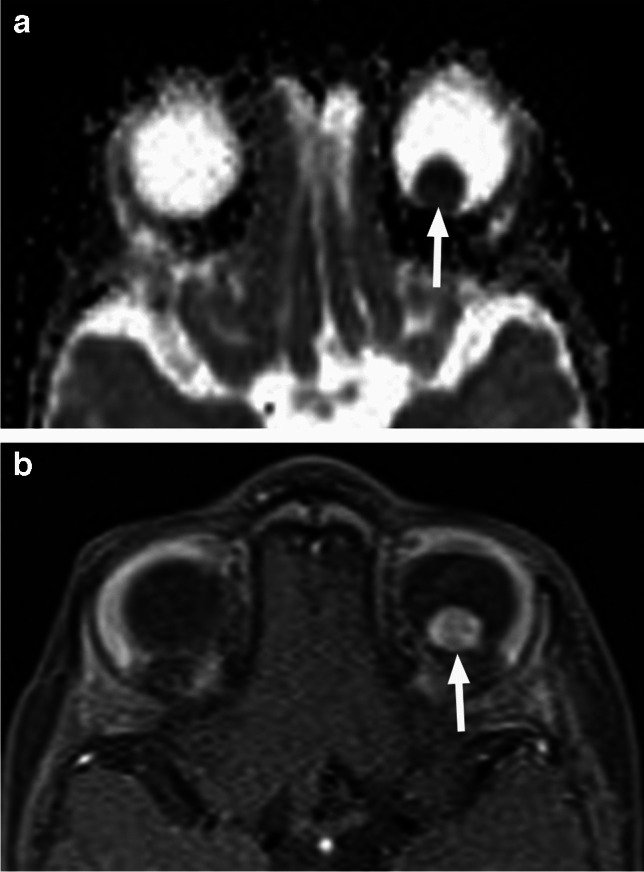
Left eye retinoblastoma in an infant. Nodular intraocular lesion (arrows) shows low diffusivity (low signal intensity, hypointense to the brain) on axial ADC map (a) and enhancement on corresponding postcontrast T1-weighted image with fat saturation (b)
